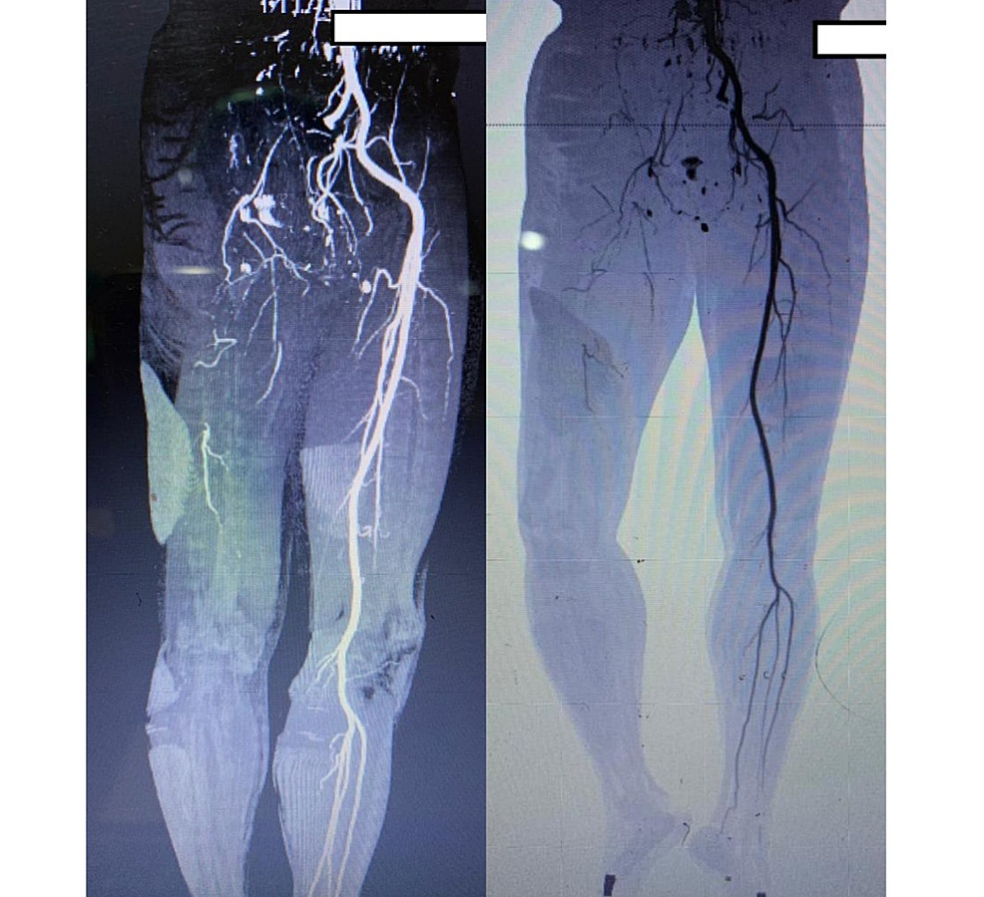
CT angiography of the lower right extremity showed no outflow from the iliac artery CT: computed tomography
